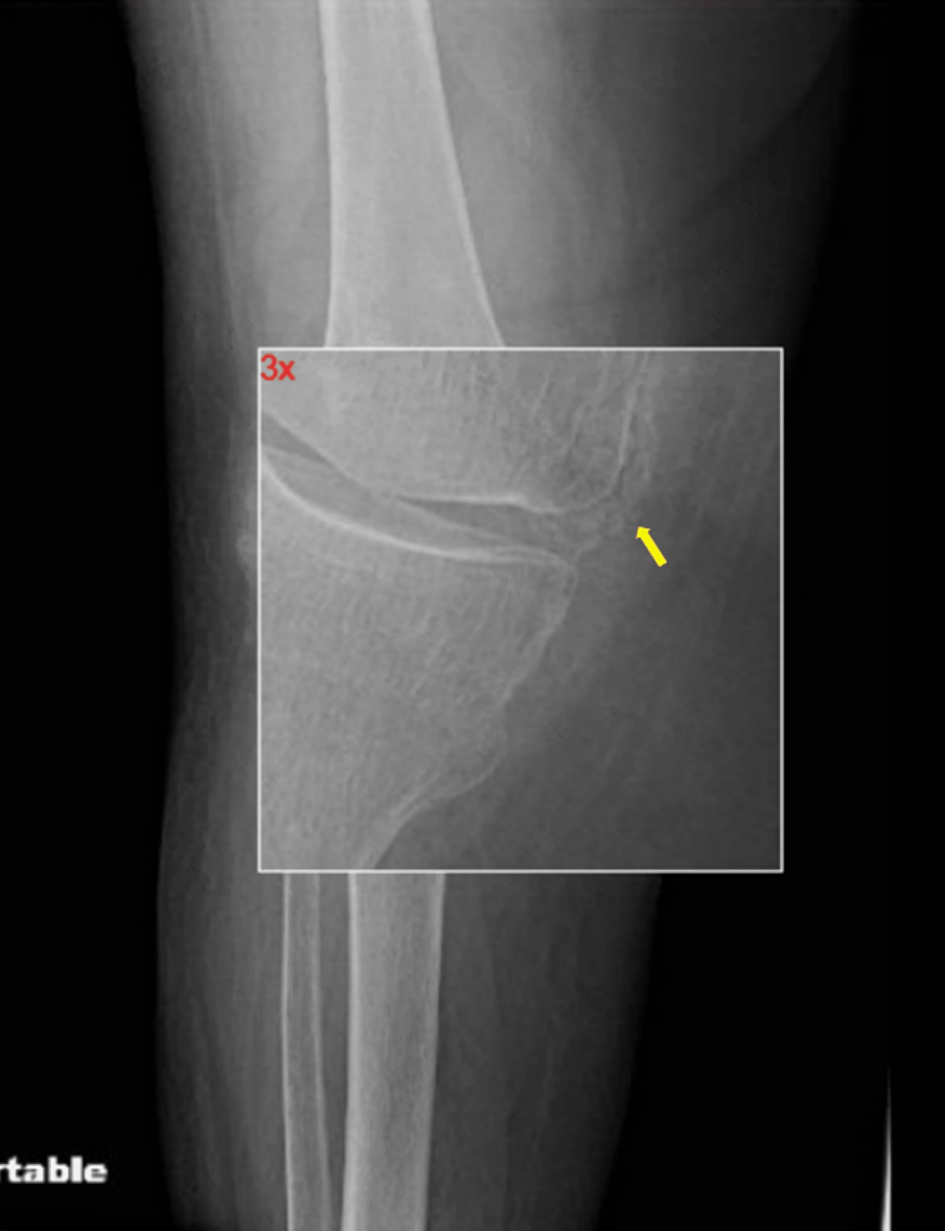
Magnified view of portable x-ray of the right knee showing chondrocalcinosis (yellow arrow)
